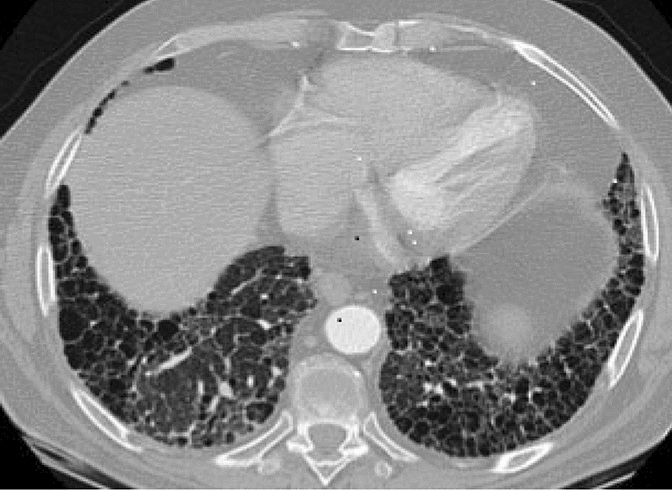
Transverse high-resolution computed tomography (CT) image of chest demonstrating a usual interstitial pneumonia (UIP) pattern—a fibrotic ILD pattern—in a patient with rheumatoid arthritis. Typically UIP pattern shows a basal predominance and emanates out from a subpleural location
